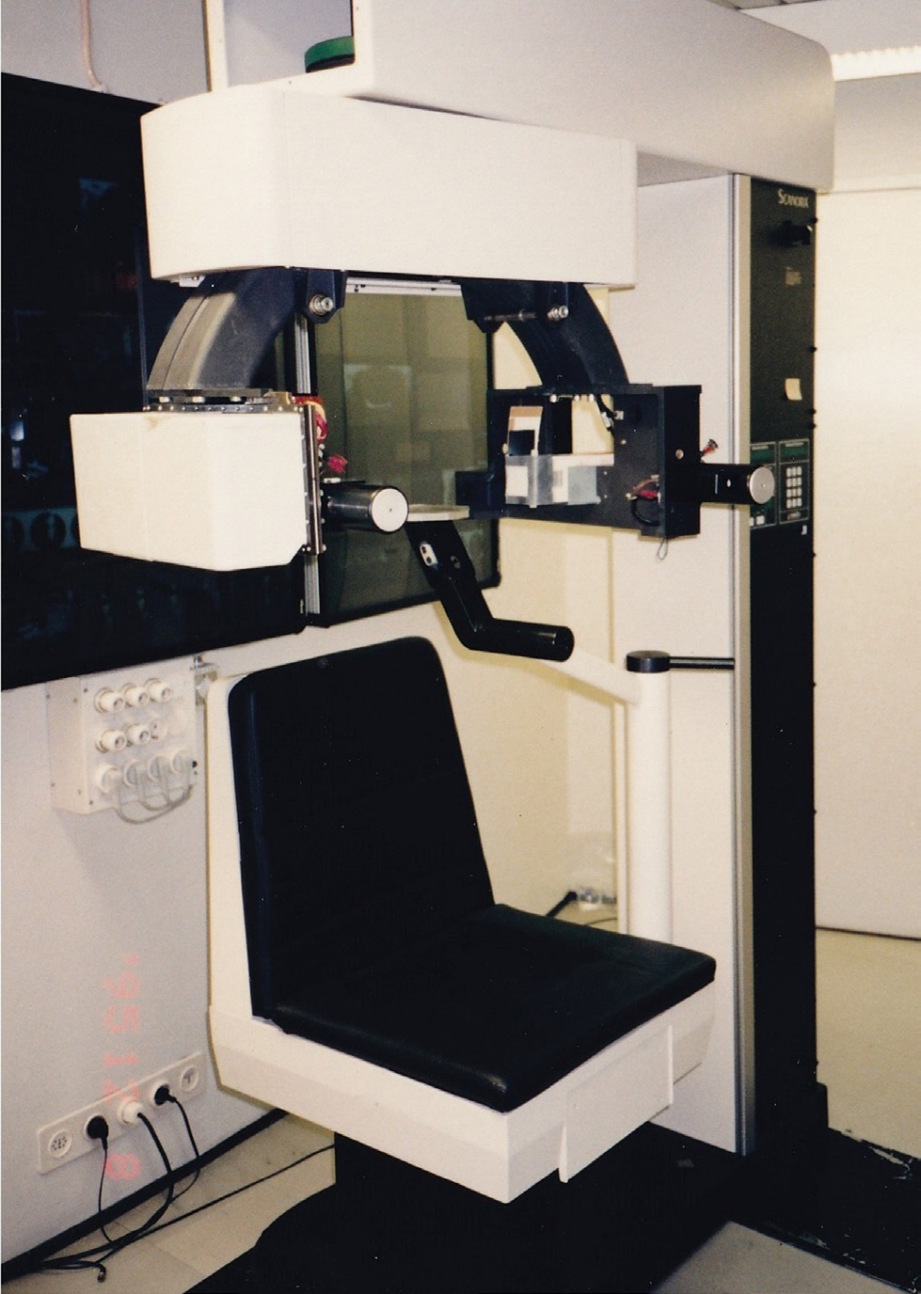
Use of *Scanora^®^* in Local cone beam CT research.
